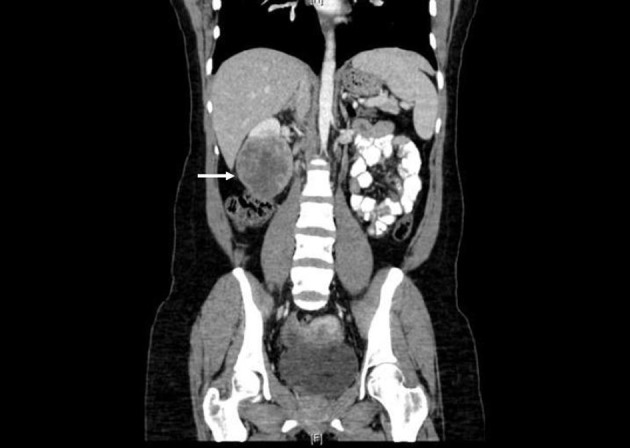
Axial CT (with oral and intravenous contrast enhancement) showing a 6.7 × 6.9 cm heterogeneous exophytic mass with areas of central low attenuation arising from the lower pole of the right kidney, with areas of central necrosis.
